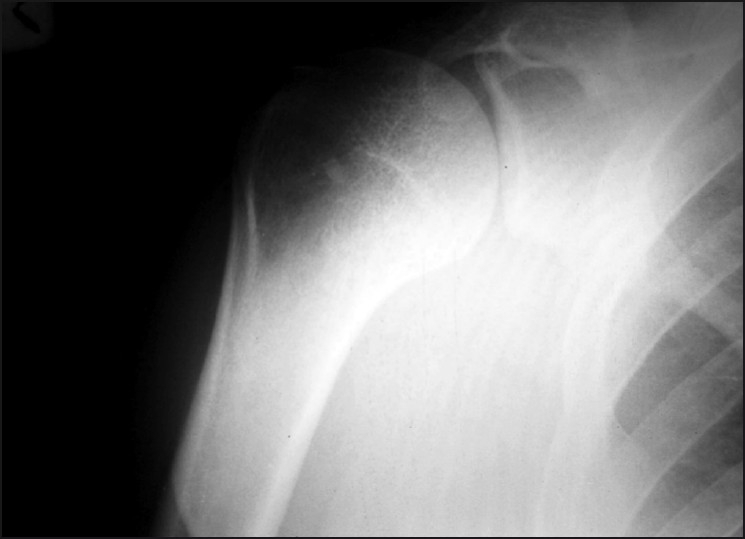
Postoperative radiograph showing absence of the calcification
